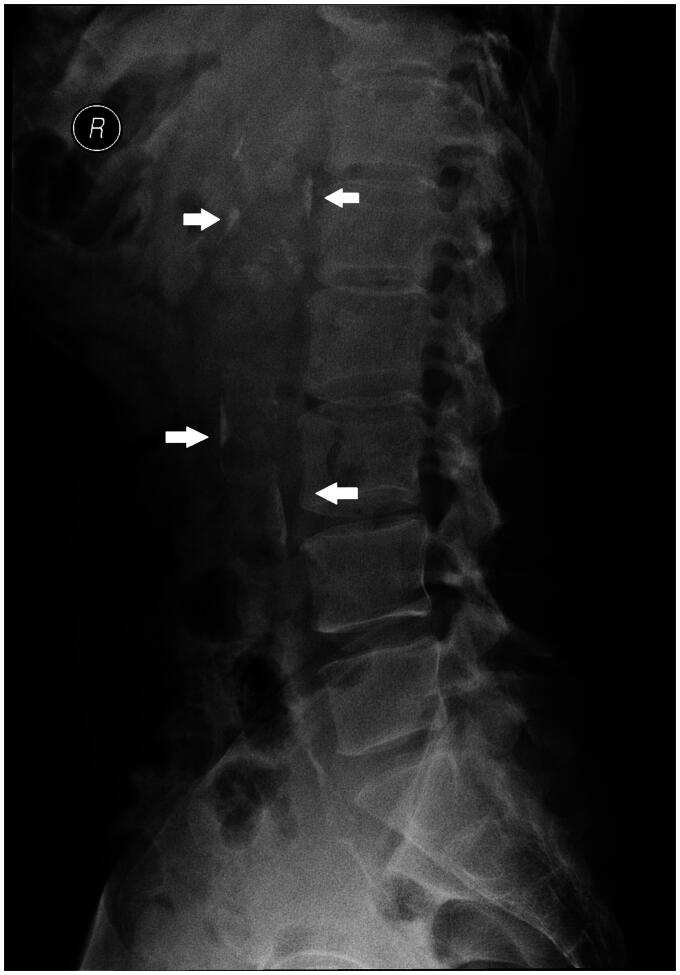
Radiological quantification of abdominal aortic calcification using abdominal lateral X-ray. Arrowhead denotes calcification sites.
